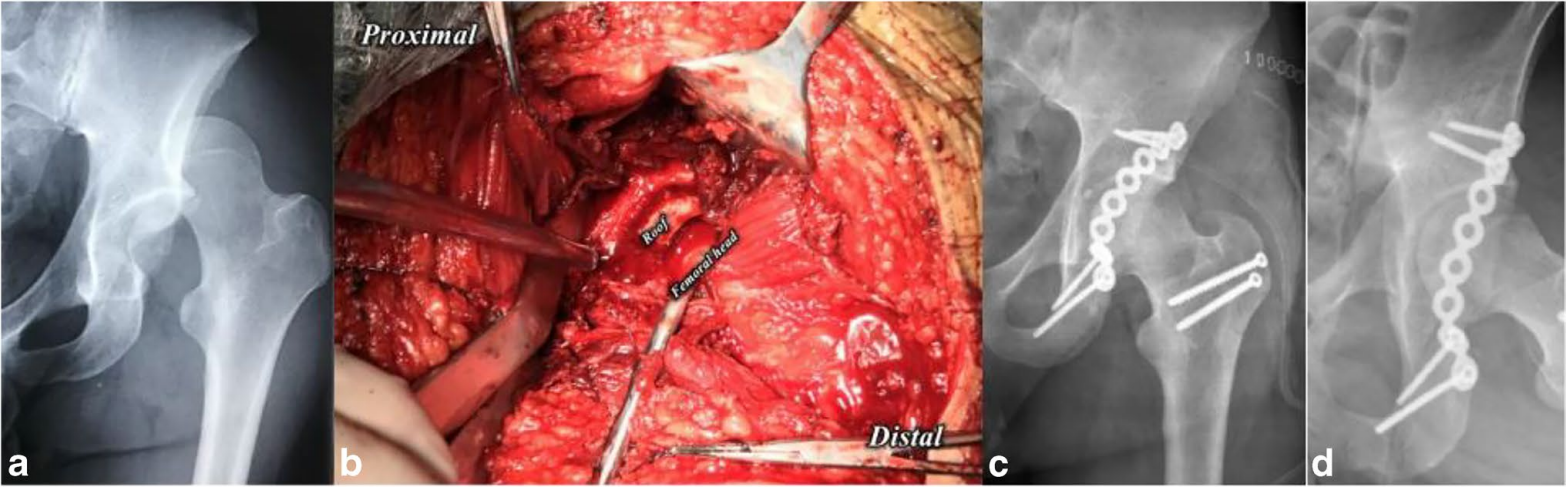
Middle-aged male with posterior wall fracture-dislocation after a traffic injury, treated via combined DTFO approach. a Left posterior wall fracture-dislocation; b Intra-operative image, DTFO help fully visualize the involved roof; c–d post-operative radiographs showing anatomic reduction
